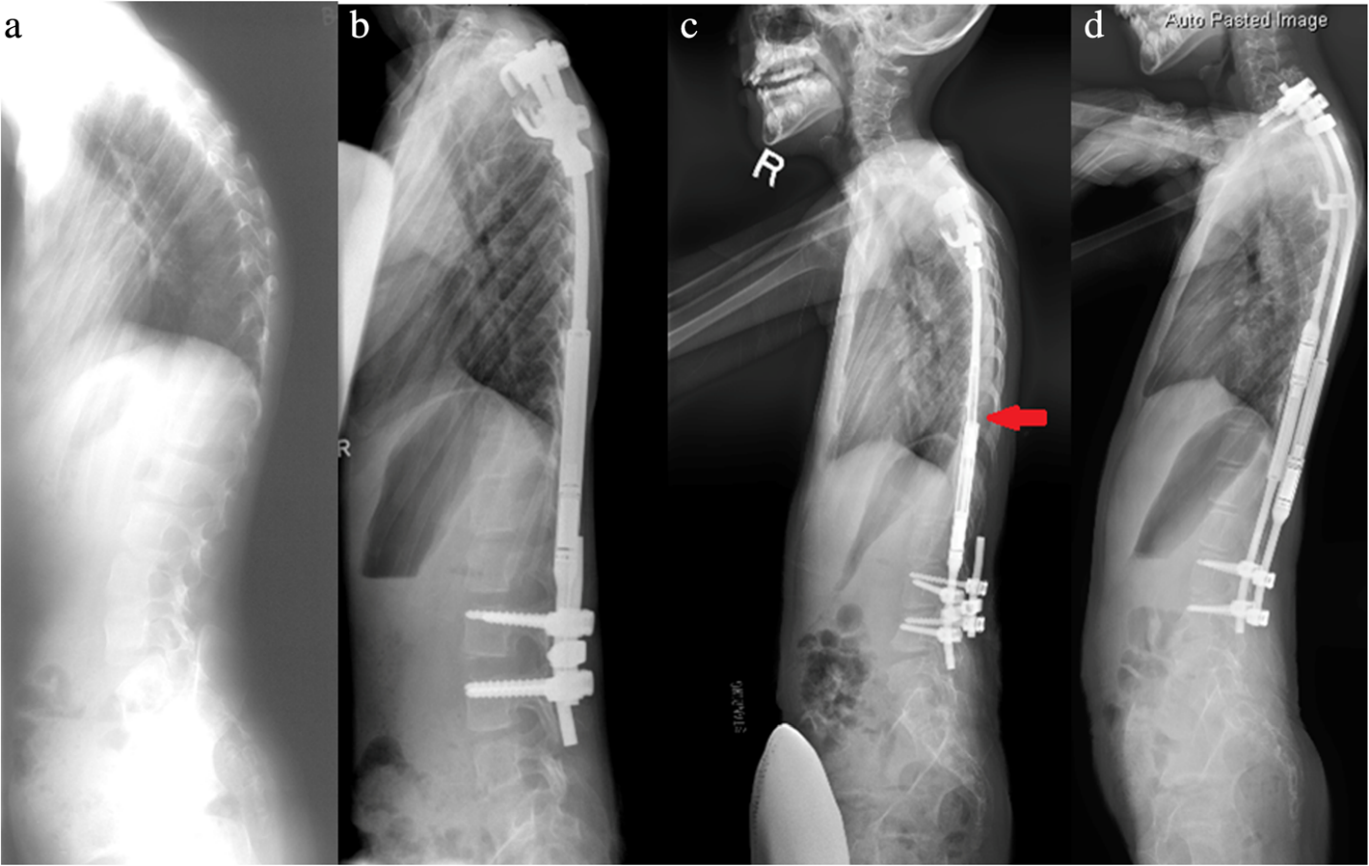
a Lateral radiographs preoperatively. b Initial single rod insertion with development of proximal junctional kyphosis after the revised rod. c A crooked rod sign in which the extendable portion of the rod is not inline with the actuator can be seen. d Extension to C7-T1 was performed
